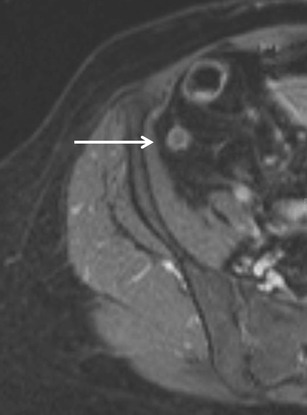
T1-weighted, fat-suppressed axial MRI after intravenous MRI contrast (gadoterate) in acute appendicitis: thickened appendix with Gd enhancement, minimal periappendiceal stranding
